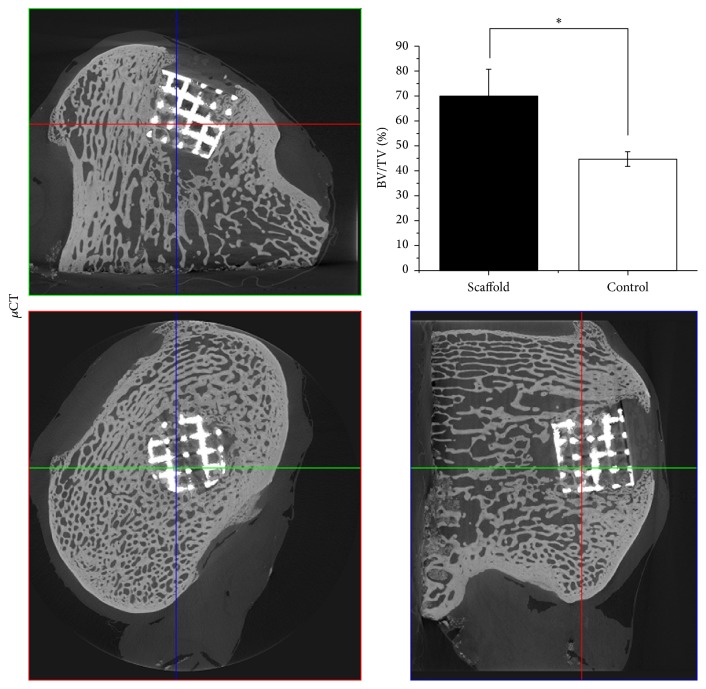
Bone ingrowth into Ti matrix. *μ*CT images show bone formation within the Ti scaffold; *∗* shows significantly higher bone volume formation in the scaffold compared to control (*p* = 0.01).
